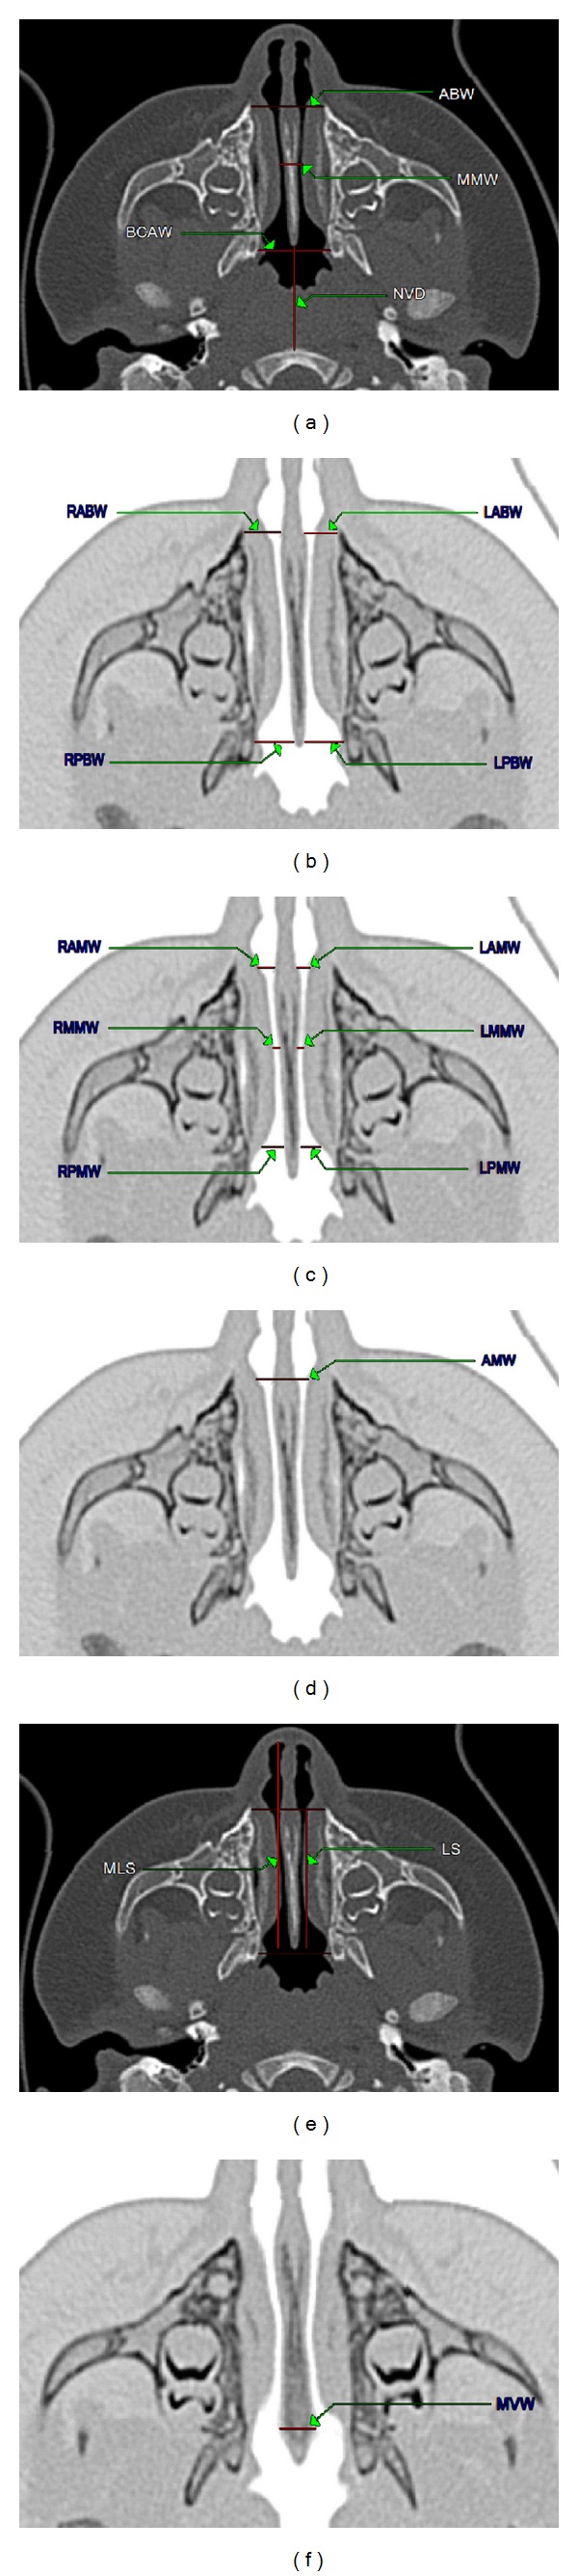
(a)–(f) Axial CT image normal nasal cavity. Measurements: (a) anterior bony width (ABW), minimal soft tissue (MMW), bony choanal aperture width (BCAW), and nasopharynx vertical distance (NVD); (b) right (RABW) and left (LABW) anterior bony width and right (RPBW) and left (LPBW) posterior bony width; (c) right (RAMW) and left (LAMW) anterior mucosal width and right (RMMW) and left (LMMW) minimal soft tissue width and right (RPMW) and left (LPMW) posterior mucosal width; (d) anterior mucosal width (AMW); (e) length of septum (LS) and maximal length of septum (MLS); (e) maximal width of vomer (MVW). Images (b), (c), (d), and (f) used color inversion.
